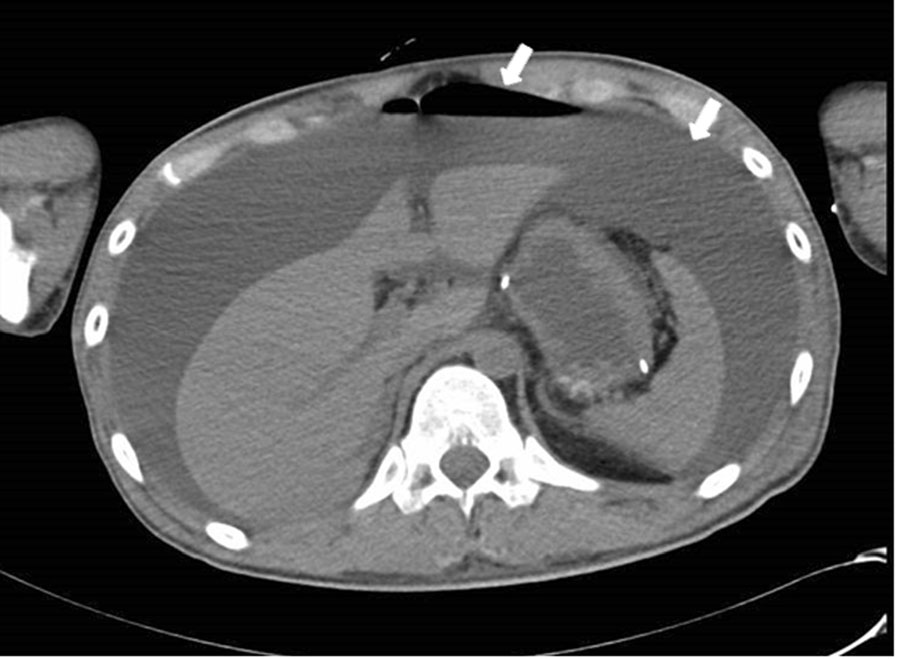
Abdominal CT. The CT scan reveals a considerable amount of fluid and free air (arrow)
